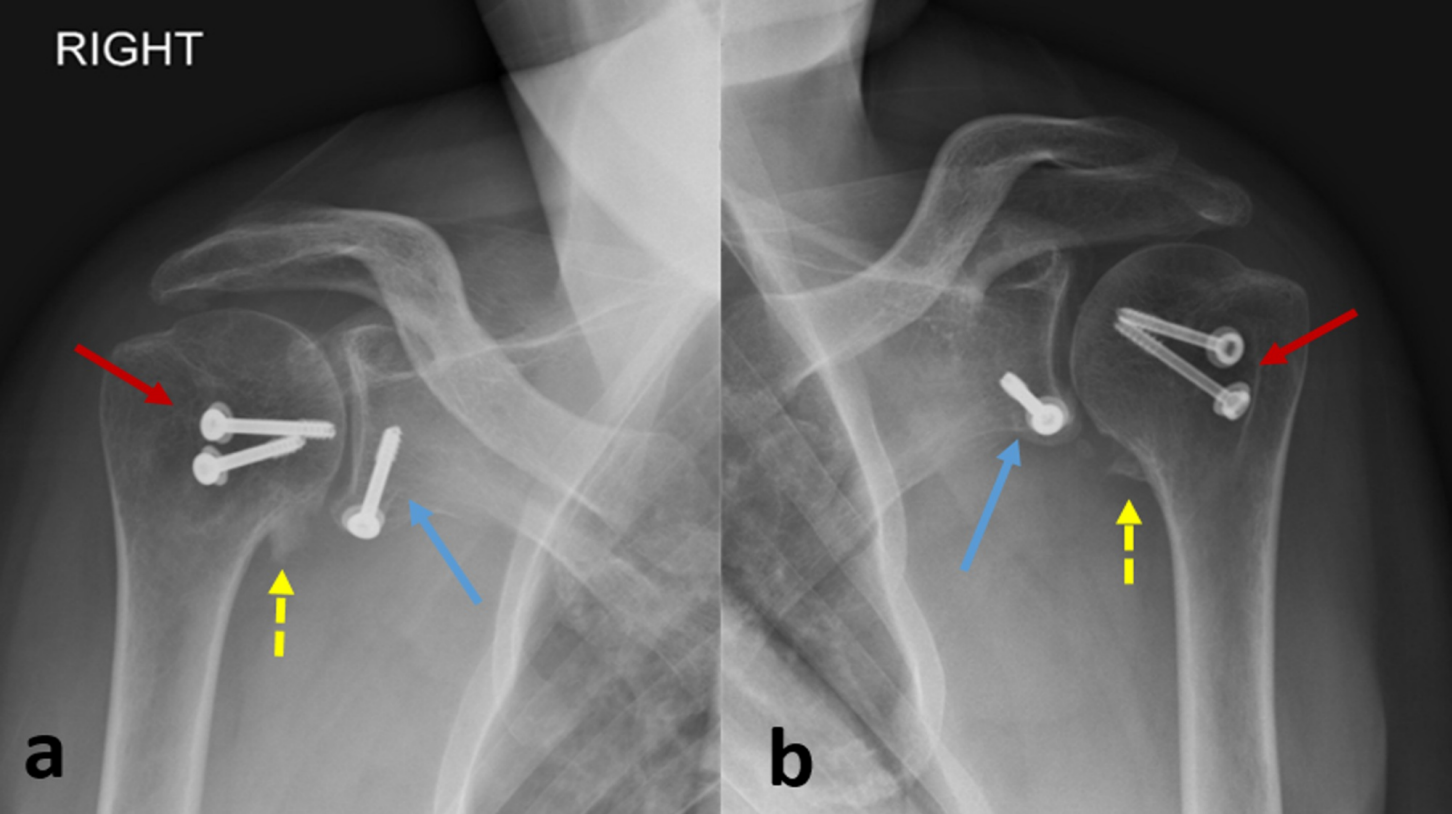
(a, b) Antero-posterior radiographs of both shoulders at 11-month follow-up: complete healing of the coracoid process to the antero-inferior glenoid rim (blue arrow), with concentric reduction of the gleno-humeral joint on both sides. Small ossification is seen at the metaphyso-epiphyseal junction of the proximal humerus bilaterally (yellow dashed arrow). The lesser tuberosity appears well reduced and healed (red arrow)
